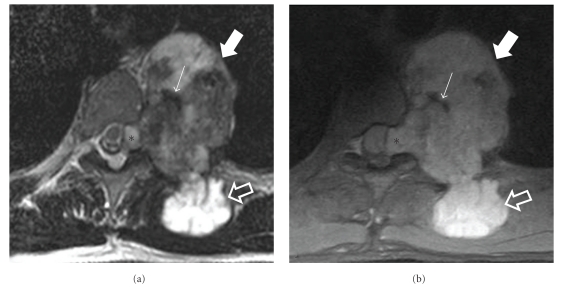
MR imaging of the same patient. The axial T2-w (a) and the axial fat-saturated PD-w (b) MR images show a heterogeneous high intensity mass (thick arrows) with mineralized elements that demonstrate low signal intensity (thin arrow). Note the superficial (open arrows) as well as the neural foraminal extension (asterisks) of the tumor.
